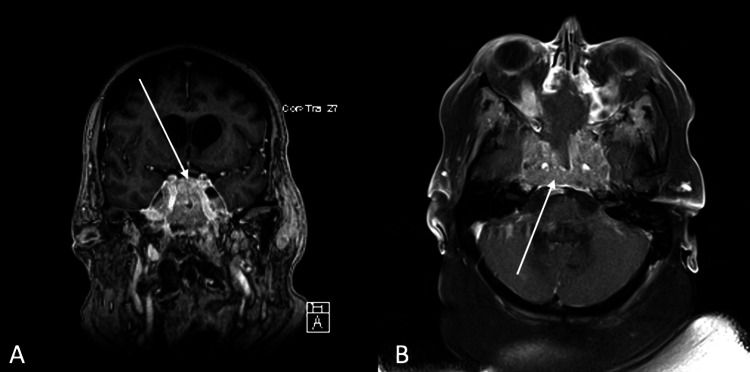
Two-month follow-up imaging. A, B: Postcontrast T1-weighted MRI demonstrating a 5.2 × 2.6 cm lesion. MRI: magnetic resonance imaging
